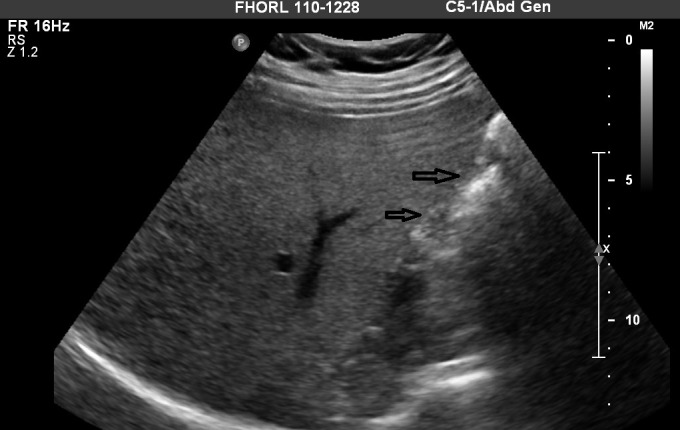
The abdominal ultrasound reported a contracted gallbladder with stones (black arrows).
